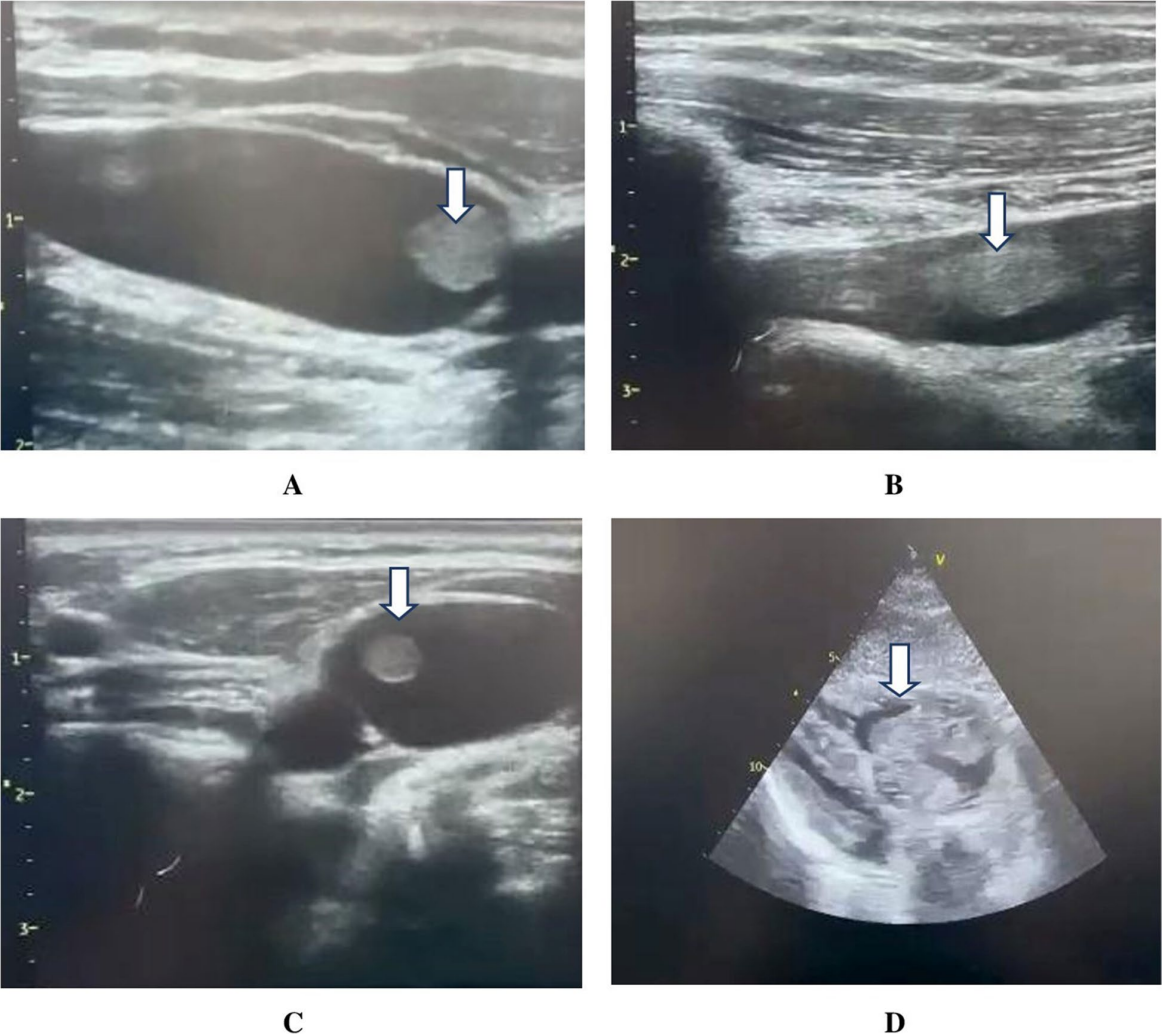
Ultrasonic findings showed a sizable thrombus (white arrow) in the pulmonary vascular (A), in the subclavian vein (B), in the right jugular vein (C), and in the right ventricular (D), respectively
